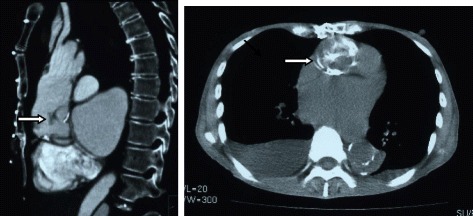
Chest CT scan before second surgery. Severe stenosis at proximal anastomosis of inner felt strip (*arrow, right*) and aortic intimal flap (*arrow, left*) (*right*, plain CT; *left*, enhanced CT)
